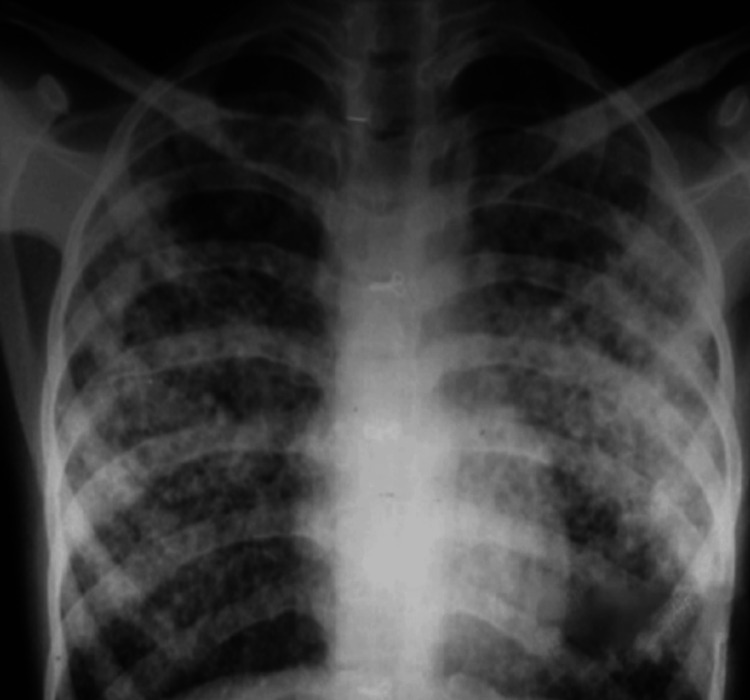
Chest X-ray showing bilateral opacities. Figure obtained from Dr. Nissar Shaikh’s image collection.
